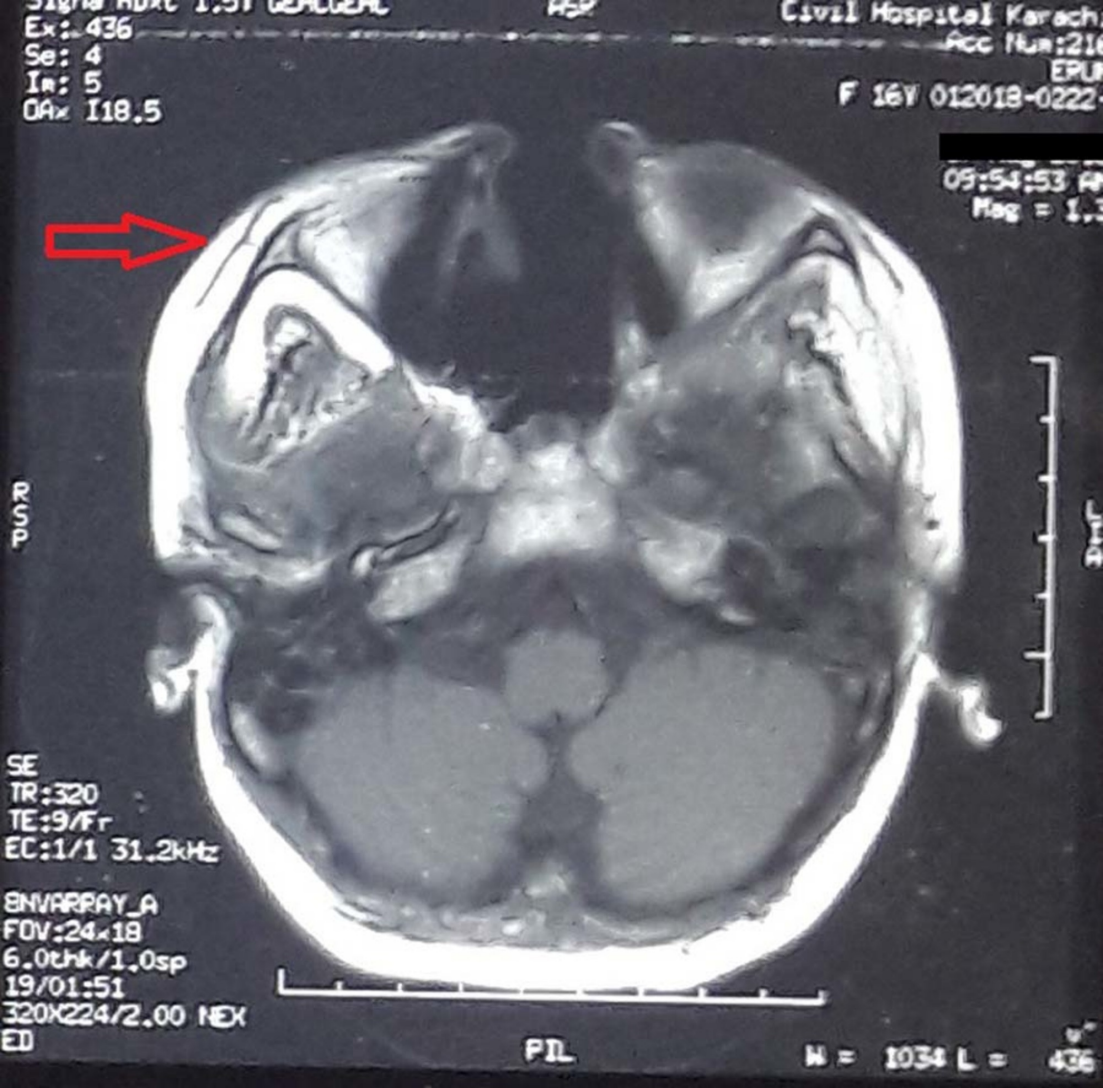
Brain MRI (axial section) T2 weighted axial images hypointense signal signifying intracranial invasion with invasive fungal rhinosinusitis.
